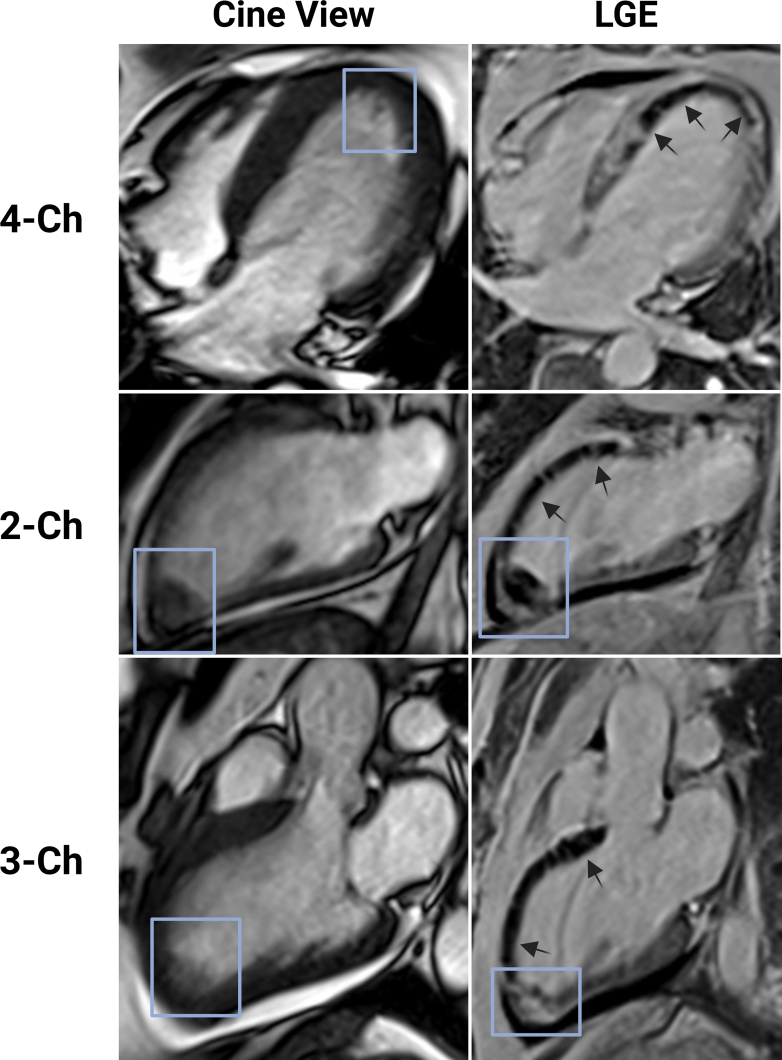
Tissue Characterization and Left Ventricular Thrombus Matched end-diastolic cine (left column) and late gadolinium enhancement (LGE) (right column) cardiovascular magnetic resonance images are shown in 4-chamber, 2-chamber, and 3-chamber long-axis views. Cine imaging reveals a distinct, mobile, low-signal-intensity mass consistent with a thrombus attached to the dyskinetic left ventricular apex (blue boxes). The corresponding LGE images demonstrate extensive transmural infarction (bright signal) of the anterior and septal walls. Black arrows indicate a substantial subendocardial zone of hypoenhancement (dark core) representing persistent microvascular obstruction, which extends to the endocardial border precisely at the site of thrombus attachment and is a sign of significant microvascular damage within the infarct zone. Ch = chamber.
